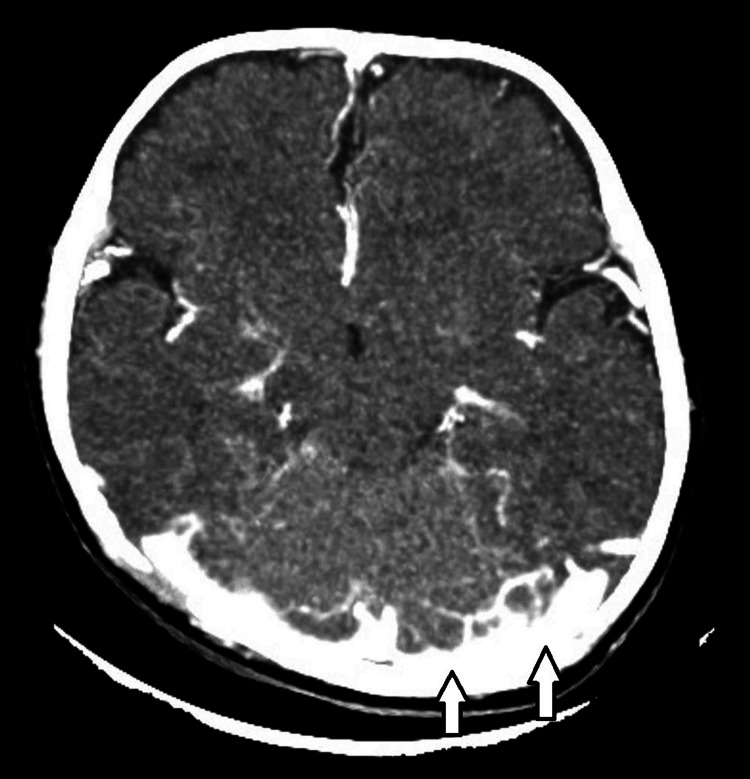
Follow-up brain CT scan showing resolution of the left-sided filling defect (normal left superior sagittal and the left transverse sinus)
